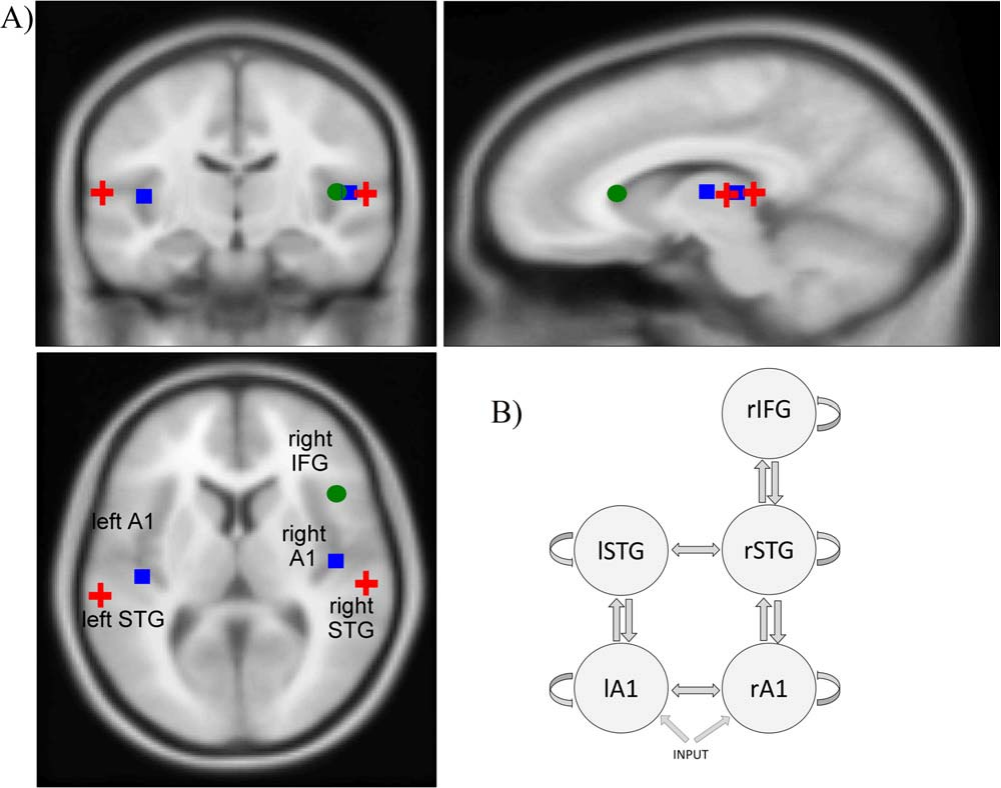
Image showing (A) the prior source locations (overlaid on an MRI image of a standard brain) and (B) the structural model used for dynamic causal modeling. The sources are linked by extrinsic (forward, backward, and lateral) connections, and each source has intrinsic inhibitory self‐connections. A1 = primary auditory cortex; STG = superior temporal gyrus; IFG = inferior frontal gyrus; l = left hemisphere; r = right hemisphere. [Color figure can be viewed in the online issue, which is available at http://wileyonlinelibrary.com.]
